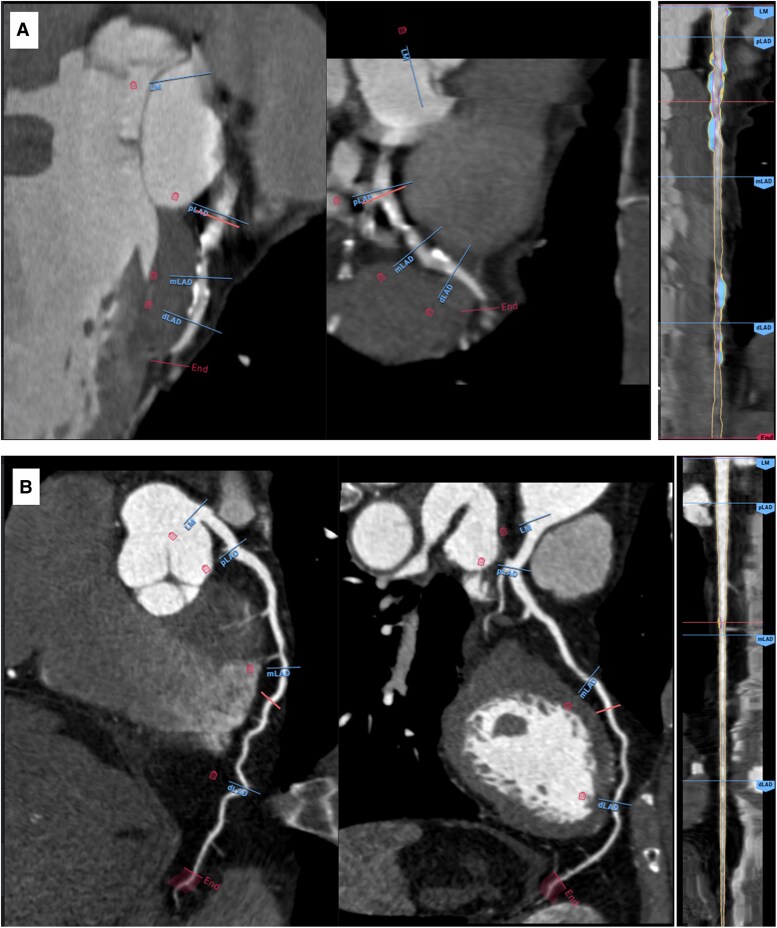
Example cases of coronary vessels analysed by AI-based quantitative computed tomography (AI-QCT). (*A*) Coronary CT angiography (CCTA) of the left anterior descending artery (LAD) from a 74-year-old man, analysed using AI-QCT, showing high PAV (34.7%) and a small ALA (2.52 mm^2^). (*B*) CCTA of the LAD from a 76-year-old man, analysed using AI-QCT, showing low PAV (9.57%) and a large ALA (4.53 mm^2^). Images used are provided courtesy of Cleerly and used with permission.
